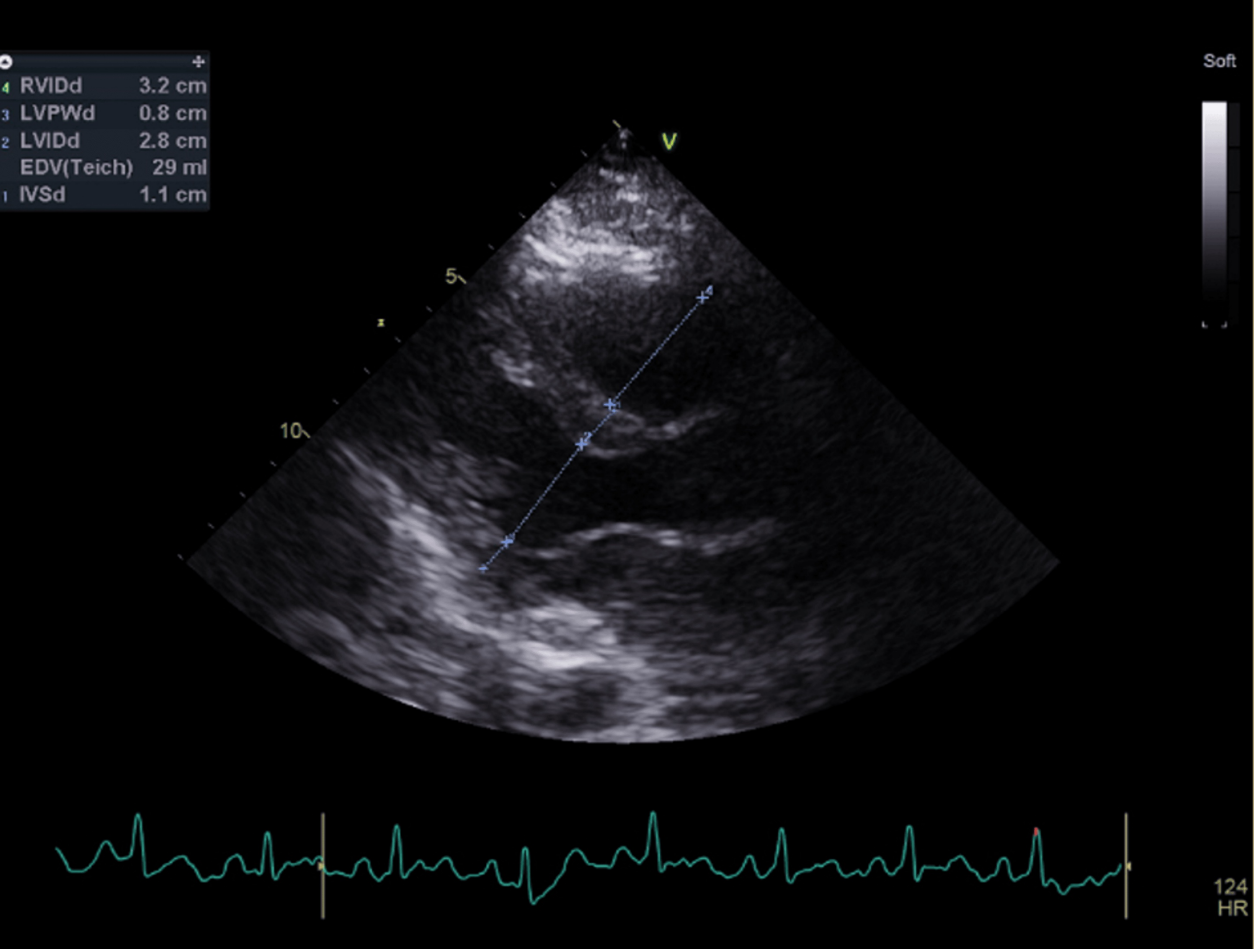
Echocardiography post pericardial drain. Echocardiogram demonstrated no significant pericardial effusion on parasternal and apical views, while subcostal views were difficult to obtain. A small amount of residual fluid was noted (<0.4 cm).
